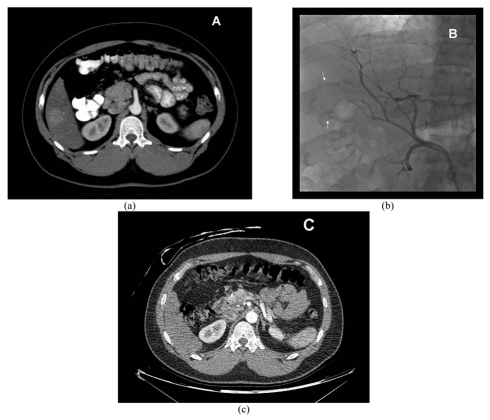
Multiphase CT in arterial phase showing a focal enhancing lesion in segment 6 (A). Angiogram before embolisation shows tumour (arrows) supplied by the branch of right hepatic artery (B). Multiphase CT in arterial phase 1 month after embolisation reveals complete disappearance of tumour, in keeping with complete response (C).
